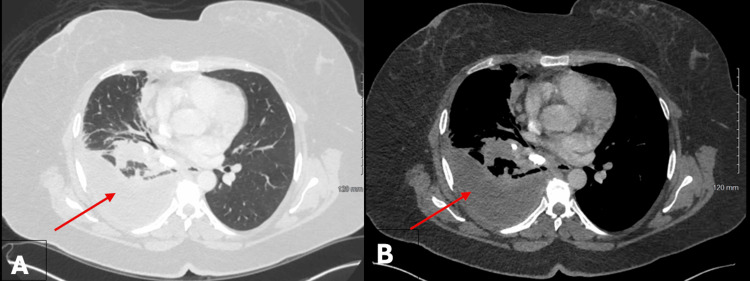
Computed tomography findings of broncholithiasis in Case 5. Axial contrast-enhanced chest CT images displayed in lung window (A) and mediastinal window (B) demonstrate a large calcified broncholith within the right lower lobe bronchus, causing complete luminal obstruction (arrows). Associated post-obstructive changes are present, with extrinsic compression of the right middle lobe bronchus.
